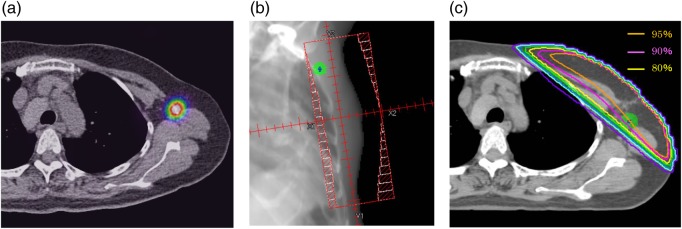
Patient example: (a) a SPECT/CT image, (b) a DRR image, (c) the dose distribution. Green circle = PTV-SLN. The SLNs are located in the middle portion of the axillary lymph node Level I. The PTV-SLN is located within the tangential field on DRR. The mean PTV-SLN dose is 92%, and the PTV-SLN D95 is 85%.
